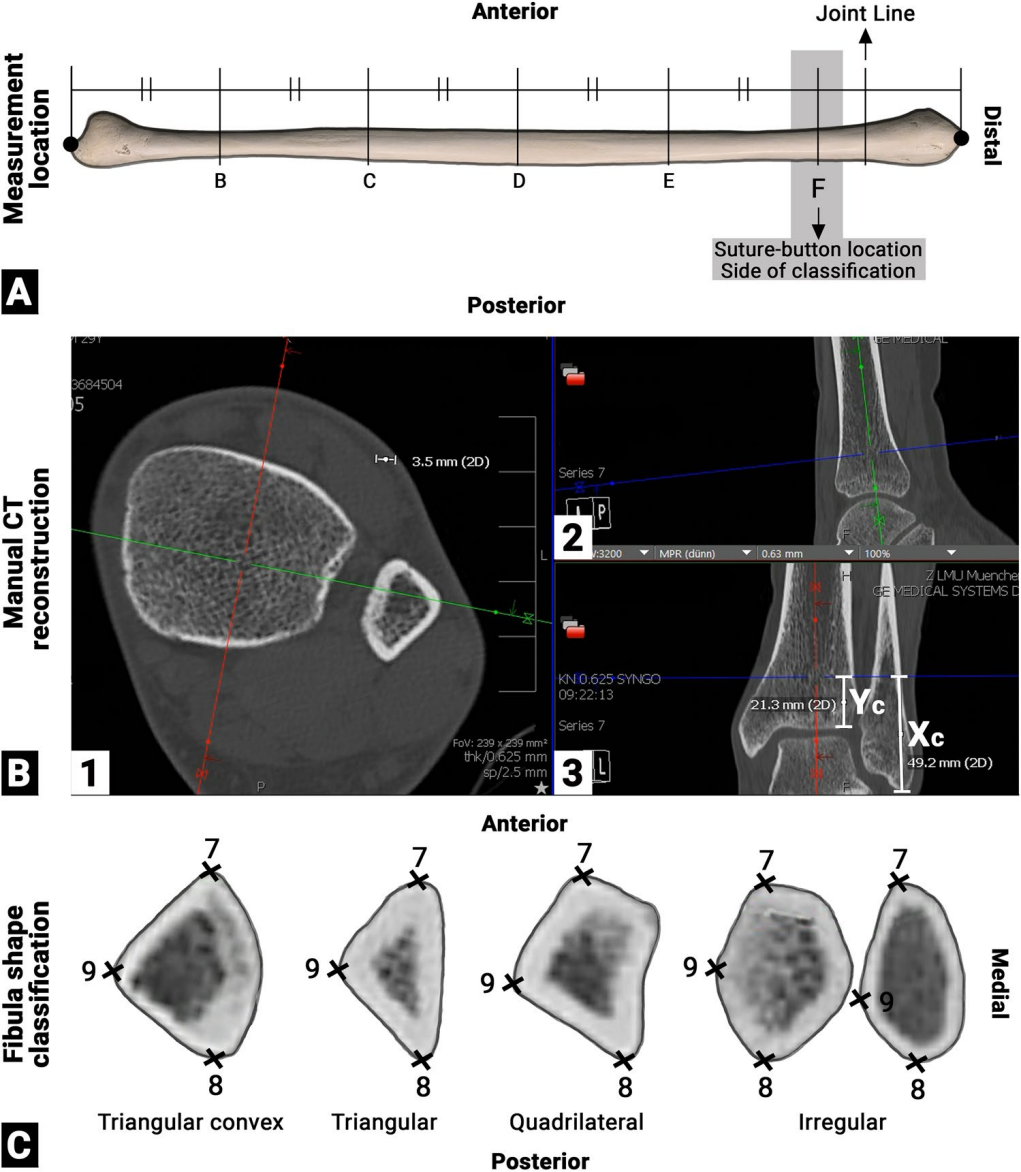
A Measurement location of the shape of the fibula B manual reconstruction of the CT datasets at the height of the suture button C different shapes of the fibula. F: measurement location for the shape of the distal fibula and the suture-button location of the contralateral, uninjured side; joint line: distal pilon tibiale; triangular convex: triangular shape with a convex hypotenuse; triangular: truly triangular shaped fibular; quadrilateral: more rectangular shaped with four edges; irregular: all other shapes, including round or pentagonal shapes; 7: anterior cortical apex; 8: posterior cortical apex; 9: lateral cortical apex; Yc: height of the measurement location from the distal lateral pilon tibial to the proximal end of the articulation between the distal tibia and fibula (suture-button location); Xc: height of the measurement location from the distal tip of the fibula to the proximal end of the articulation between the distal tibia and fibula (suture-button location)
